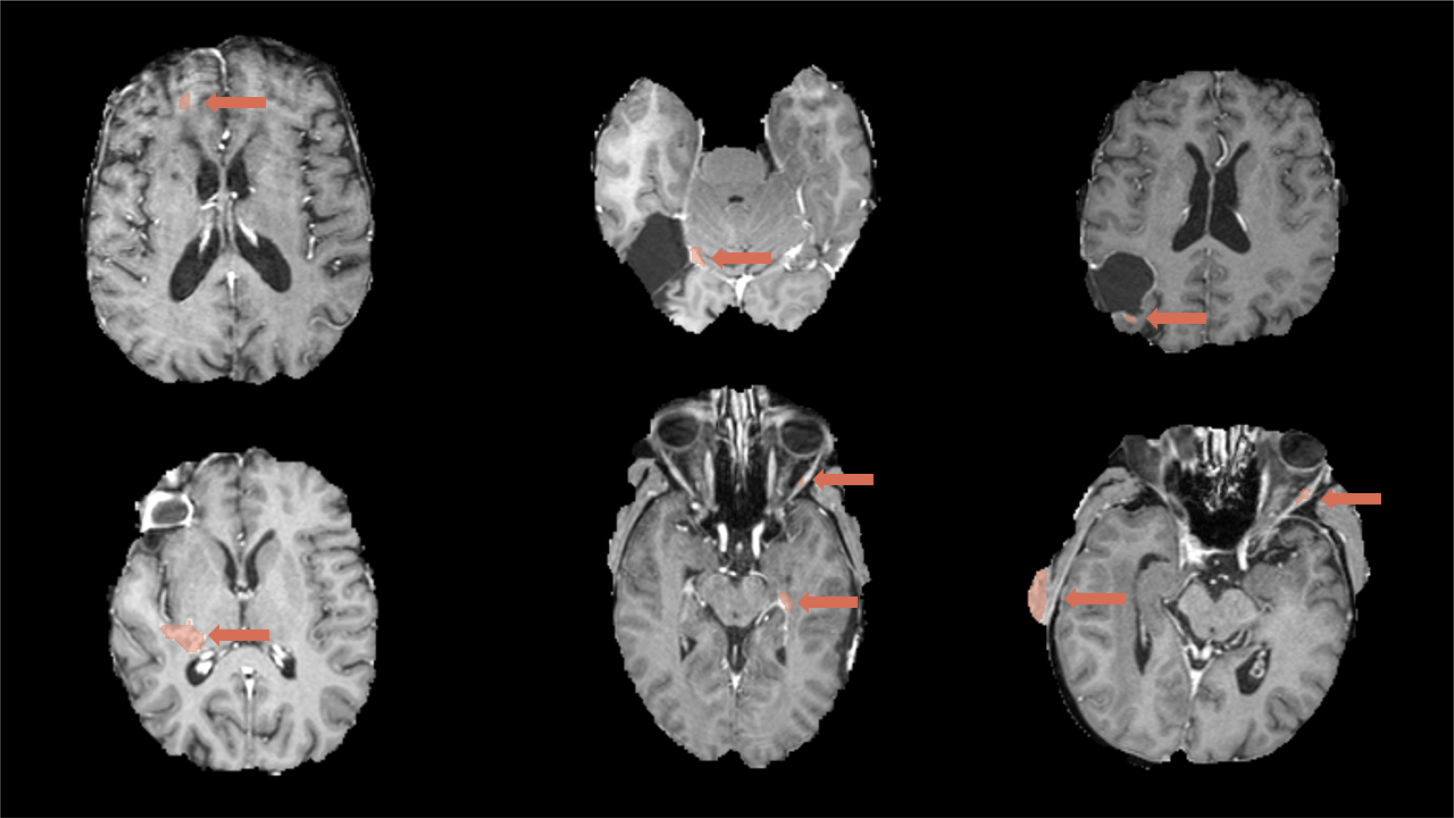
Examples of false-positive segmentations by BraTumIA that were removed during the manual correction, shown together with the post-contrast T1-weighted image. Top left: false positives potentially caused by image artifacts; top middle and right: false positives along the resection cavity; bottom left and middle: distant false positives including vessels; bottom middle and right: false positives caused by incomplete skull-stripping.
